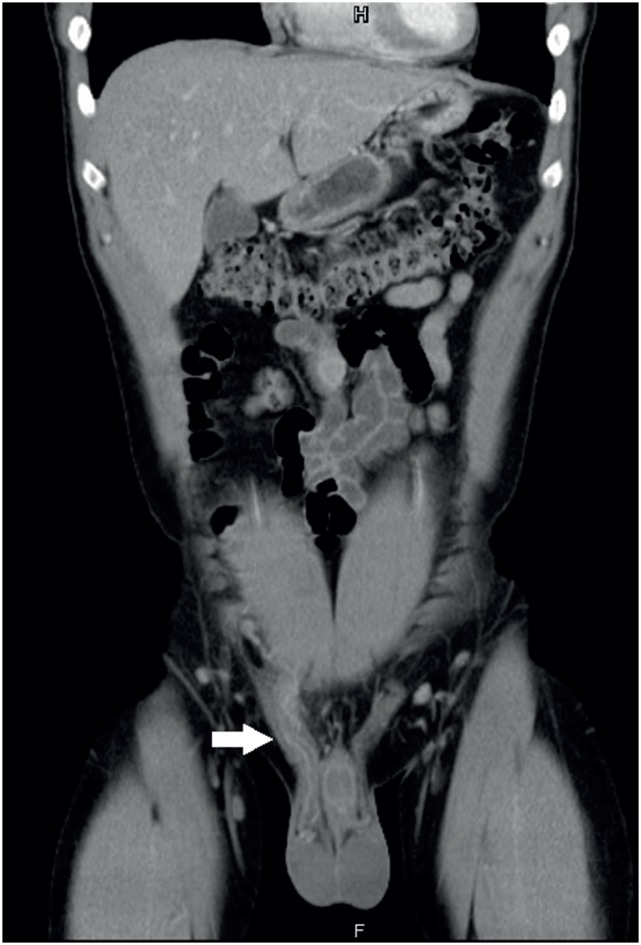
Prominent thickening of the right spermatic cord as compared to normal left spermatic cord on coronal computed tomography.
